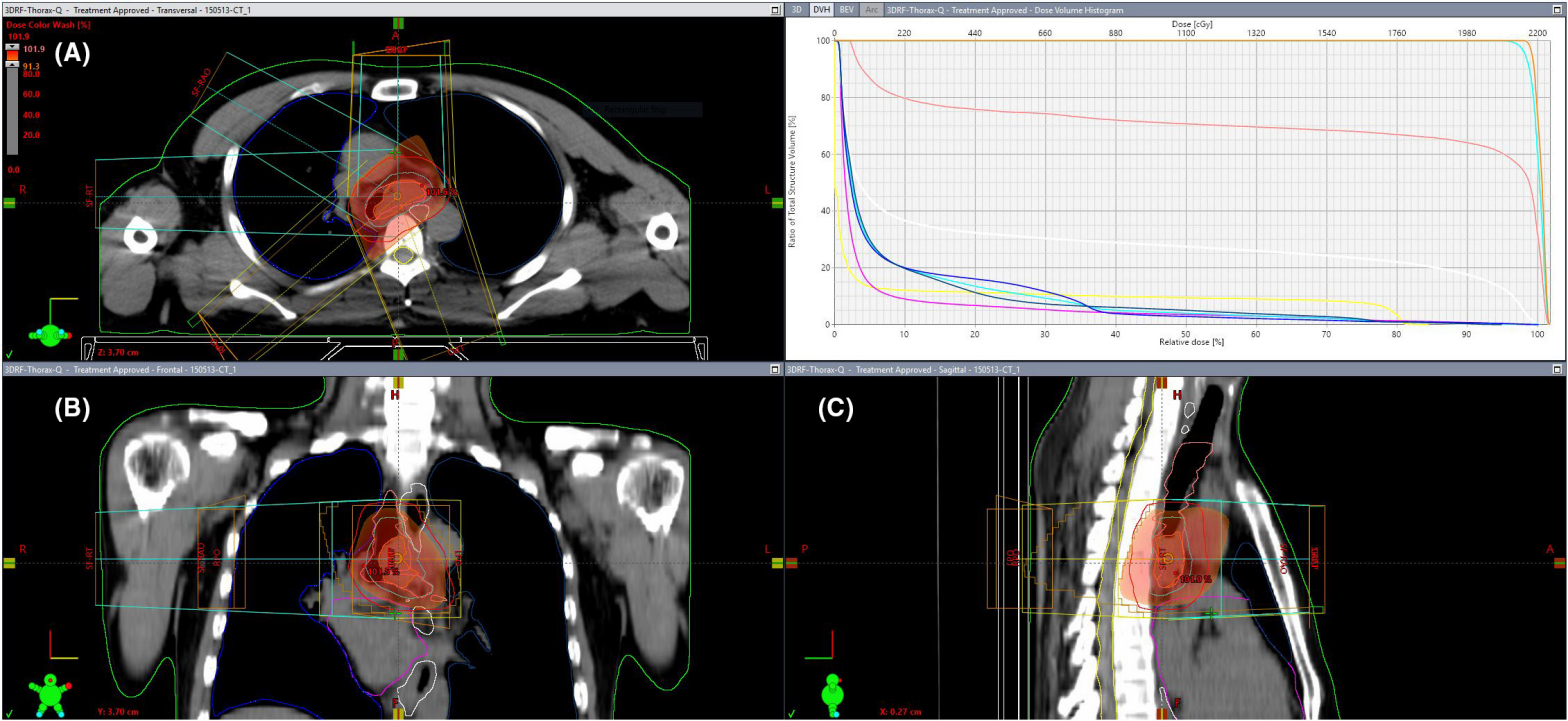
Radiotherapy plan presented in computed tomography scans of the treated regions with 3D reconstruction. Isodose levels are shown in the dose color wash method around 6600 cGy (red line) and DVH (dose volume histogram). (A) Axial view; (B) Sagittal view; (C) coronal view.
